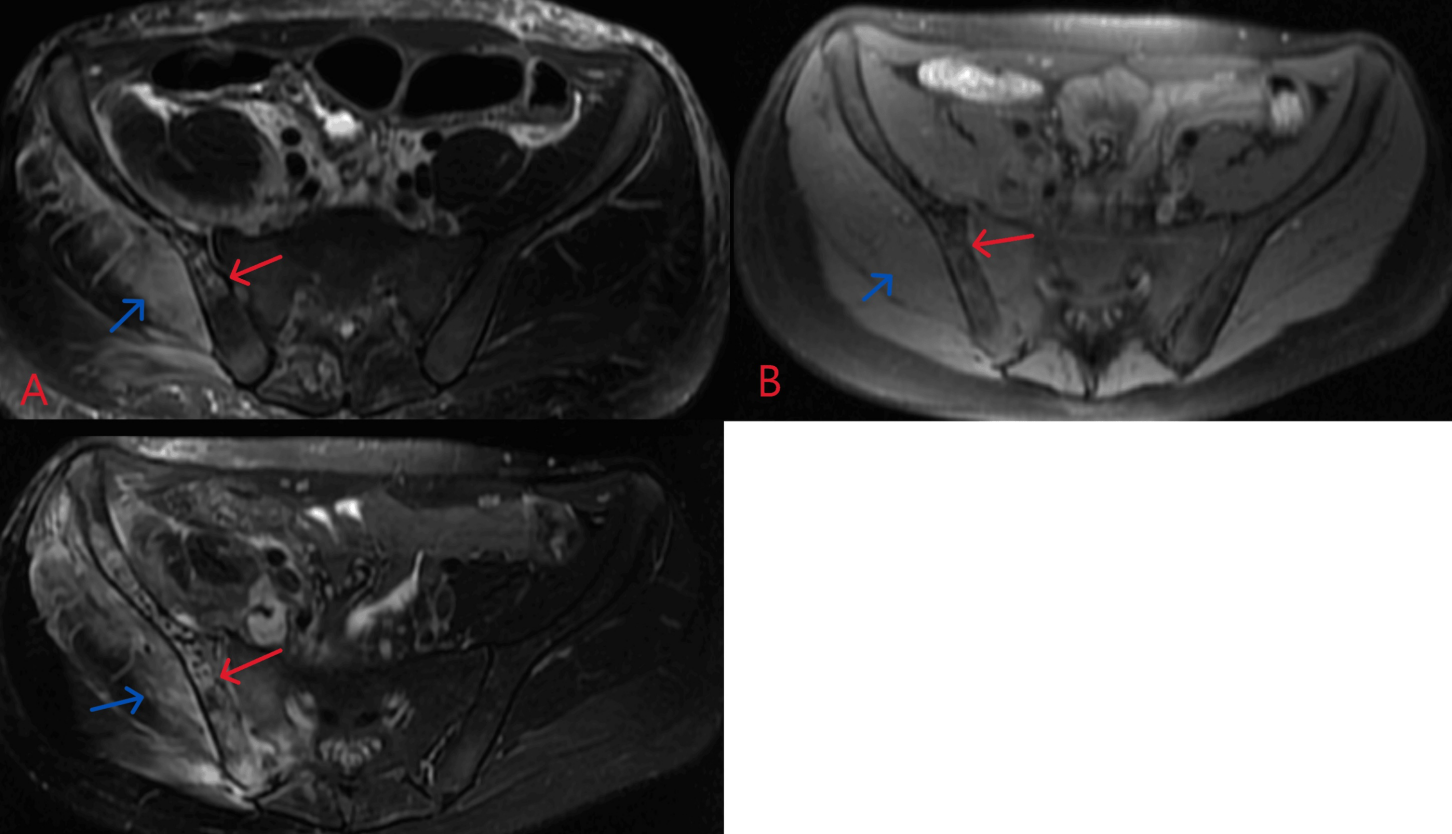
Follow-up MRI pelvis two weeks after initial presentation. T1 pre-contrast (A), T1 post-contrast (B), and T2 (C). Within the right iliac bone, there is a progression of the previously seen heterogeneous decreased T1 signal, increased T2 signal, and lack of postcontrast enhancement within the iliac bone consistent with advanced osteomyelitis with osteonecrosis (red arrows). There is similar myositis throughout the musculature of the right hemipelvis and hip region (blue arrows).
